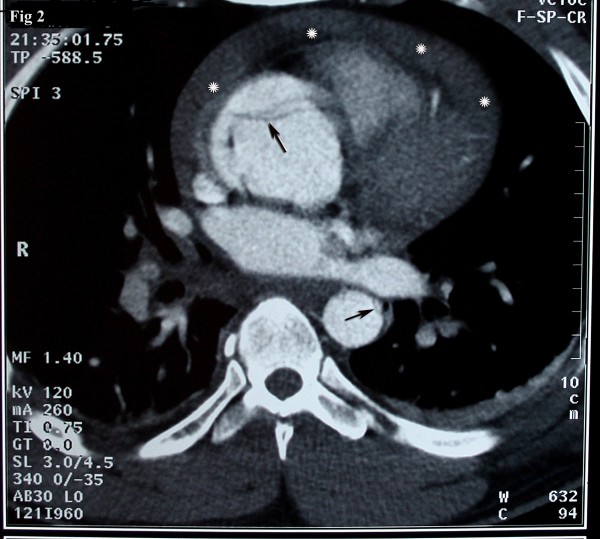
Axial source image of CT angiography reveals the flap in the ascending aorta compatible with type A of Stanford dissection: Arrows are showing the flap in ascending aorta and astrixes are showing hemopericardium.
